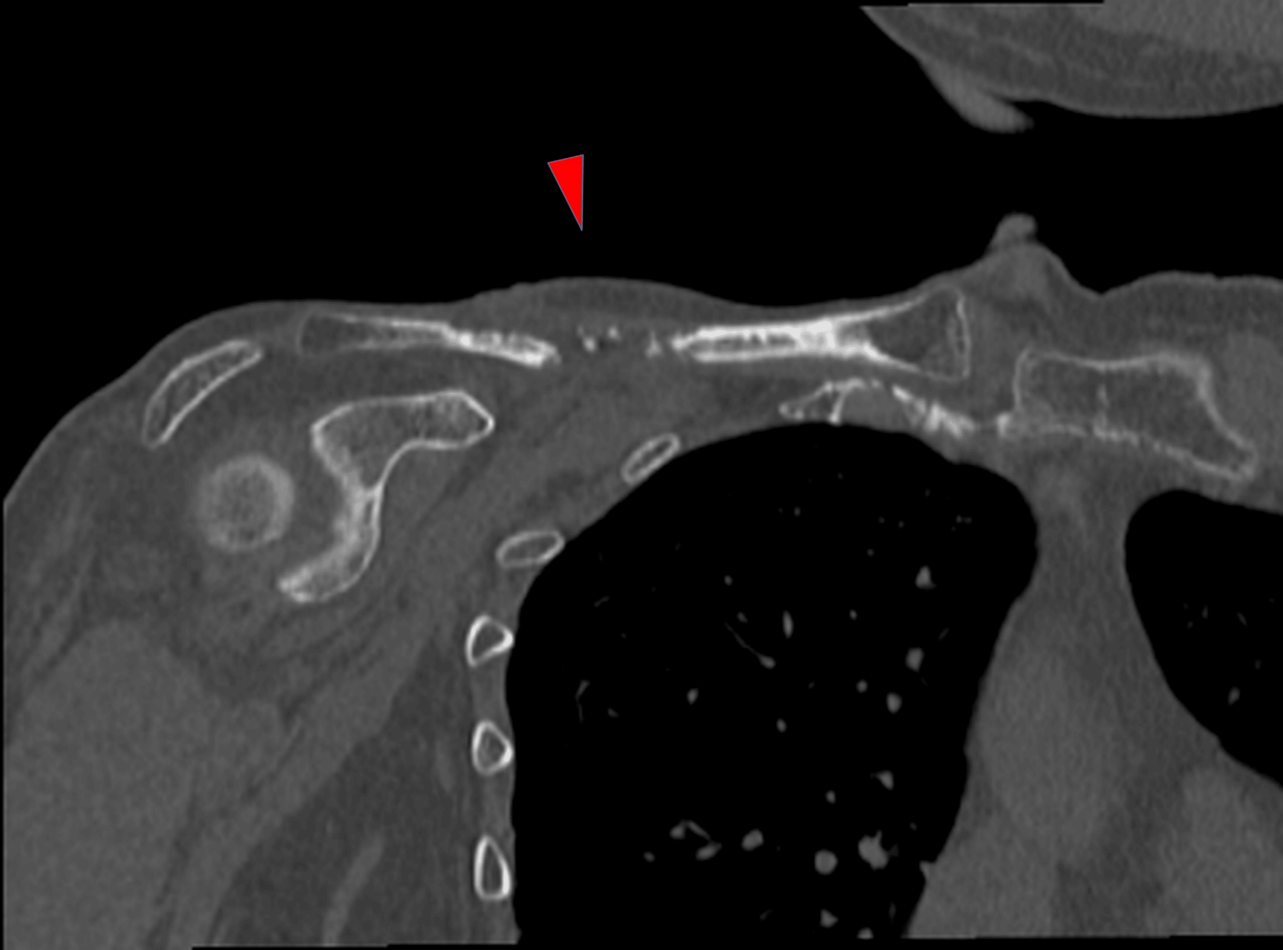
Coronal CT image of the upper limb. Clavicular fracture demonstrated without evidence of osteolytic changes (arrow).
